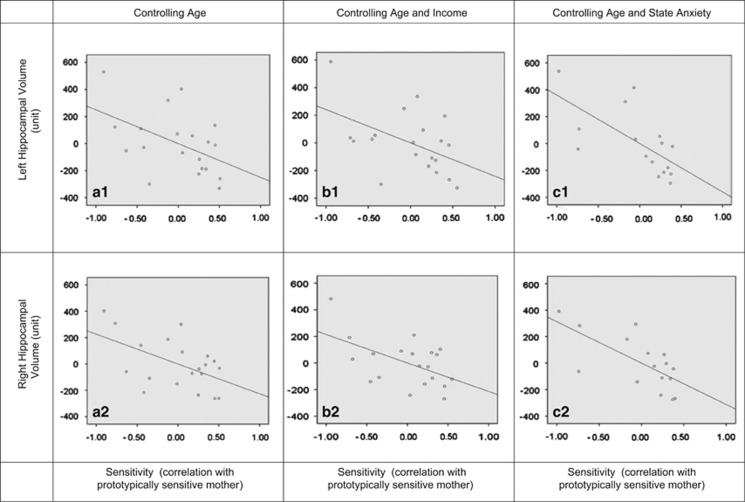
Partial regression plots of the association between maternal sensitivity and hippocampal volume. (a1) The association between maternal sensitivity and left hippocampal volume, controlling for age at MRI. (a2) The association between maternal sensitivity and right hippocampal volume, controlling for age at MRI. (b1) The association between maternal sensitivity and left hippocampal volume, controlling for age at MRI and household income. (b2) The association between maternal sensitivity and right hippocampal volume, controlling for age at MRI and household income. (c1) The association between maternal sensitivity and left hippocampal volume, controlling for age at MRI and maternal postnatal state anxiety at 3 months. (c2) The association between maternal sensitivity and right hippocampal volume, controlling for age at MRI and maternal postnatal state anxiety at 3 months. MRI, magnetic resonance imaging.
